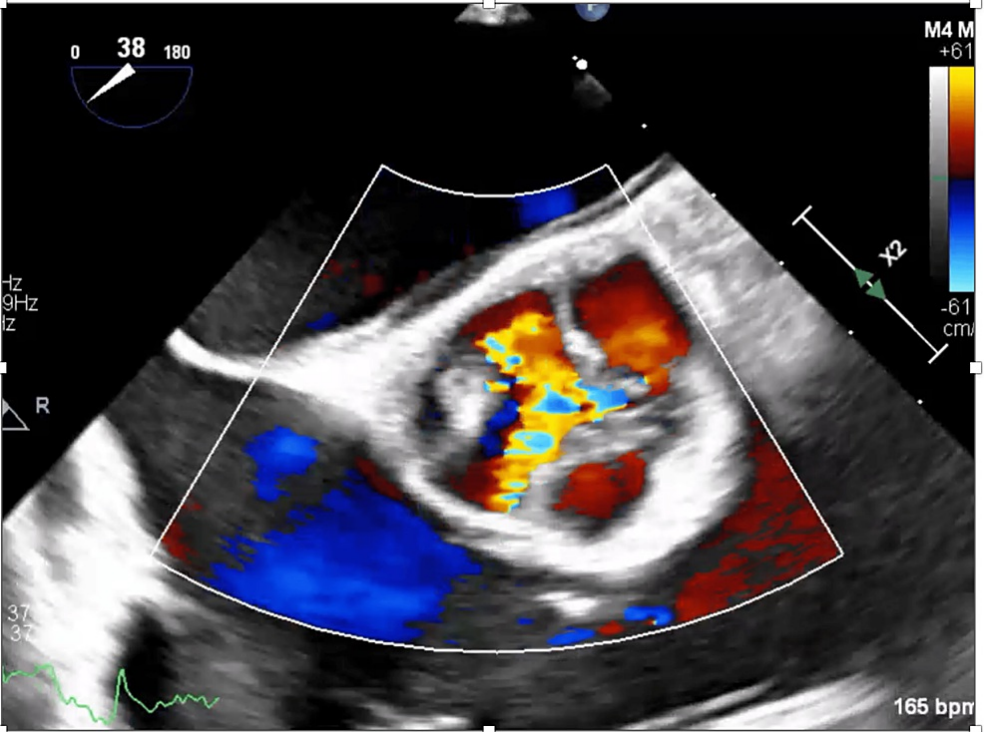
Color Doppler trans-esophageal echocardiogram showing severe aortic insufficiency
